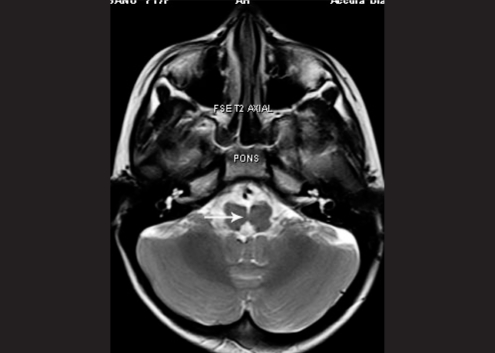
MRI brain of case 1, T2 axial, arrow denotes pontine cleft (split pons sign) (c) MRI spine of case 1, T2 coronal
